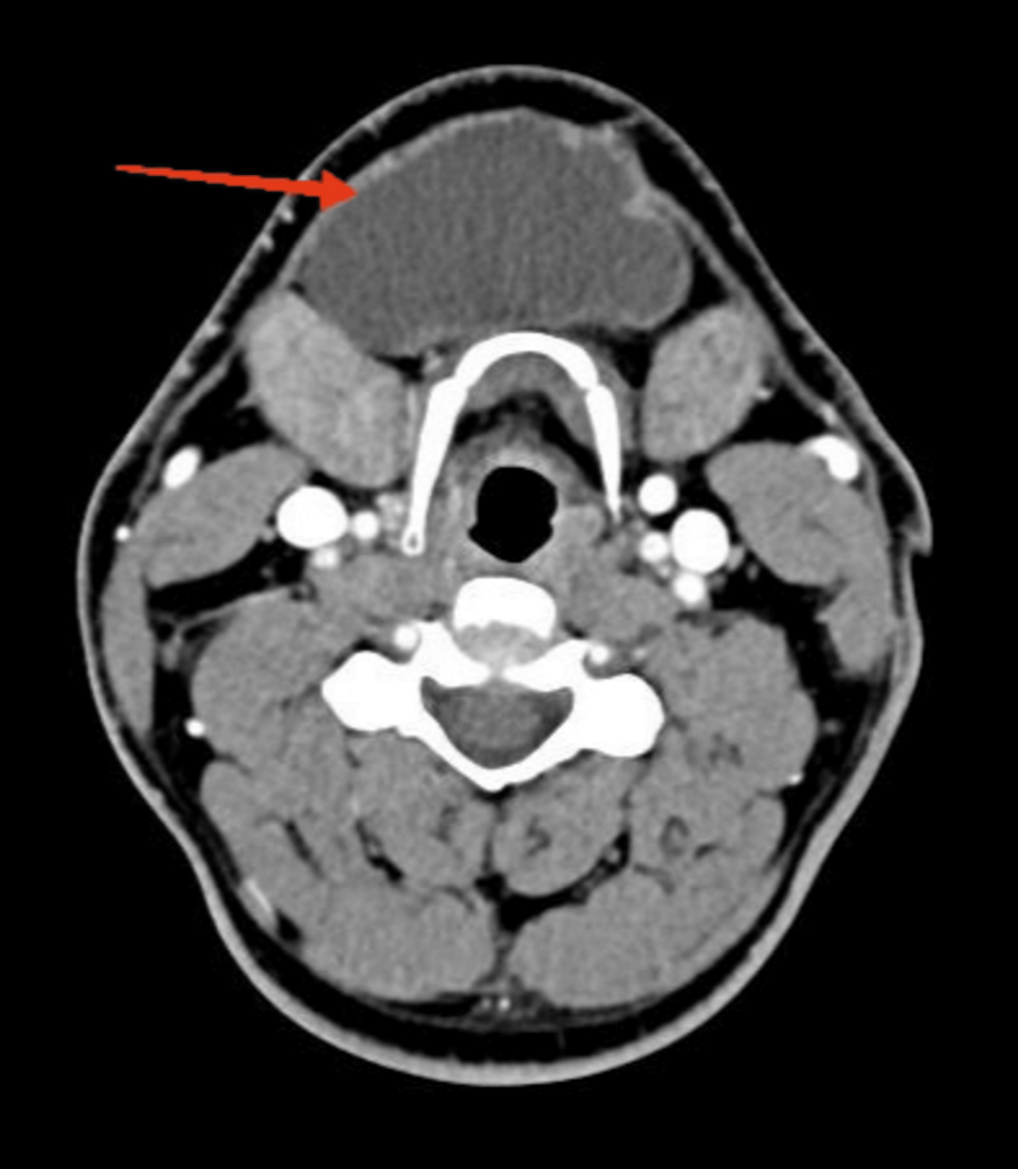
Sagittal section of the CT with intravenous administration of contrast material, showing a fluid-equivalent mass well demarcated from the surrounding parenchyma, without peripheral enhancement. It is located medially in the submental space, in close proximity to the right sublingual gland, without communication with the left sublingual gland CT, computed tomography
